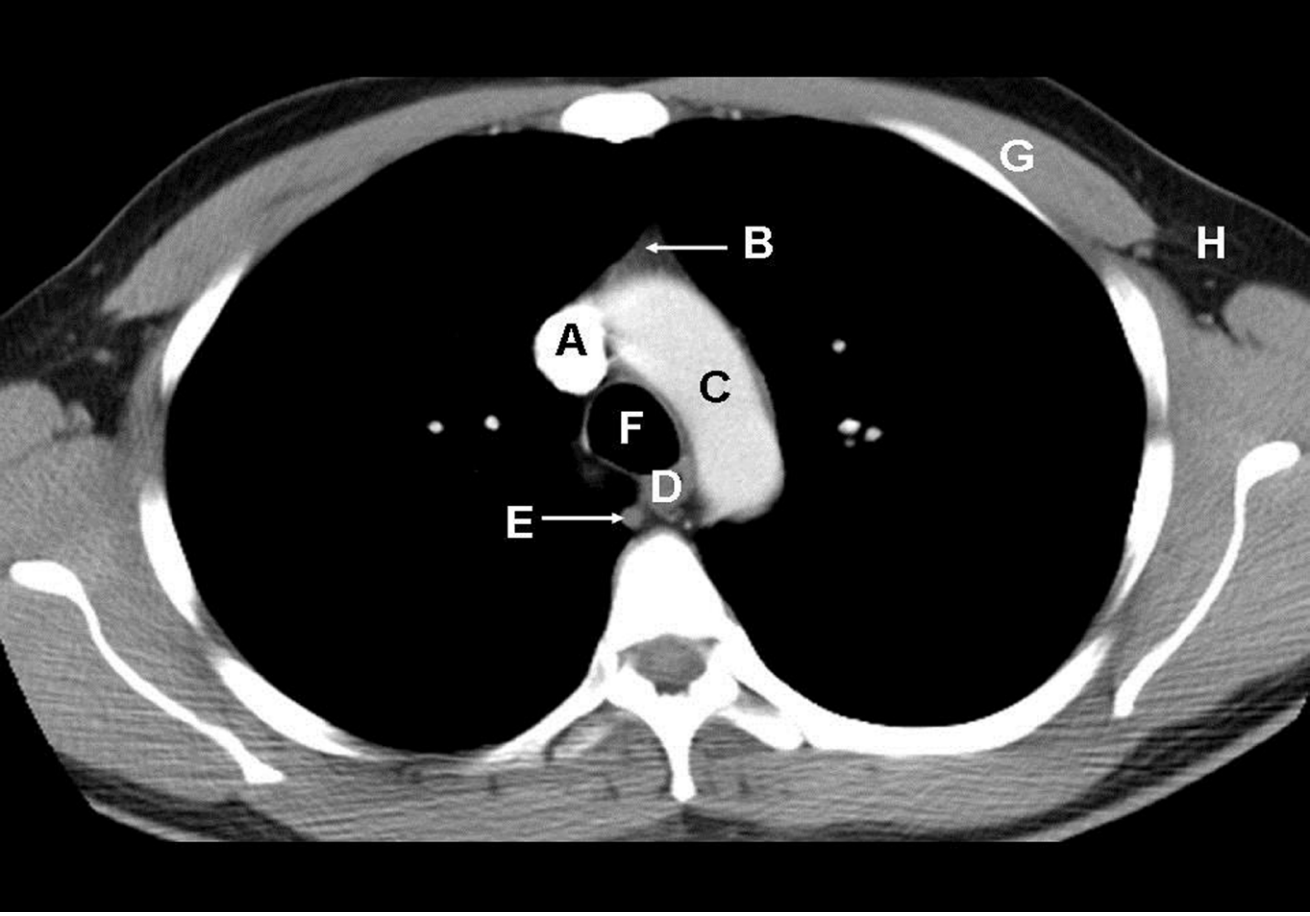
On the postcontrast-enhanced axial CT image of the chest of a 27-year-old man shown above, identify the labelled normal anatomical structures.
